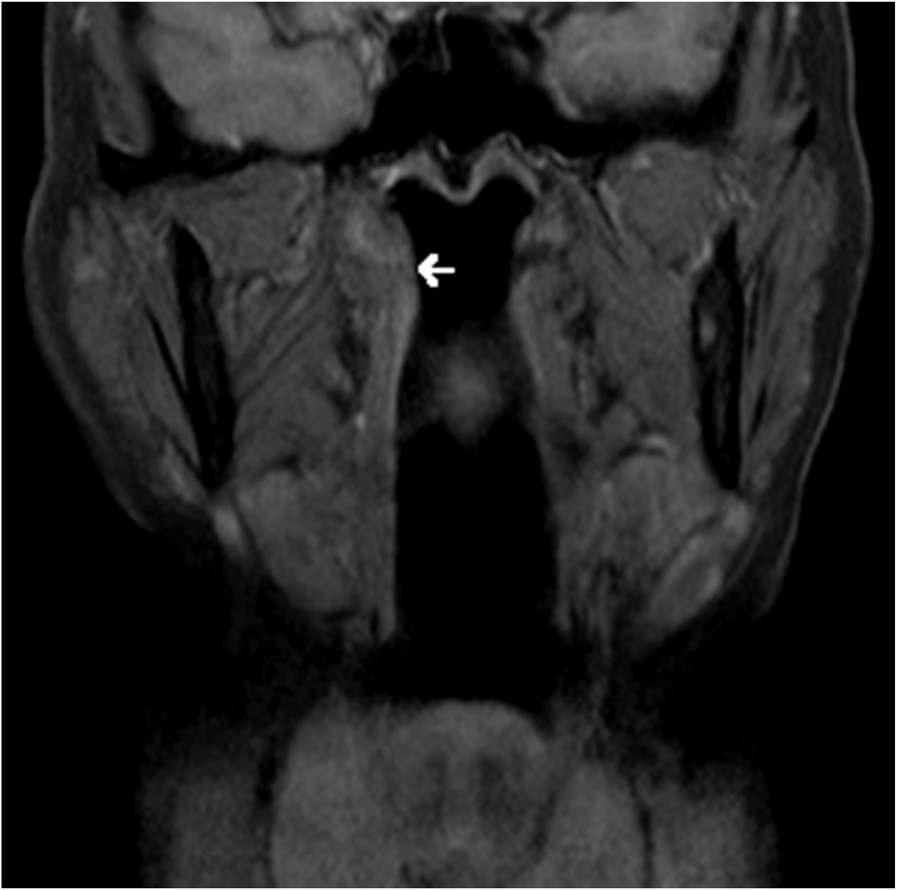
T1-weighted coronal MRI image demonstrating thickening of the right Eustachian tube orifice(arrow). The lesion appears limited to the Eustachian tube and nasopharynx, without invasion of adjacent structures.
